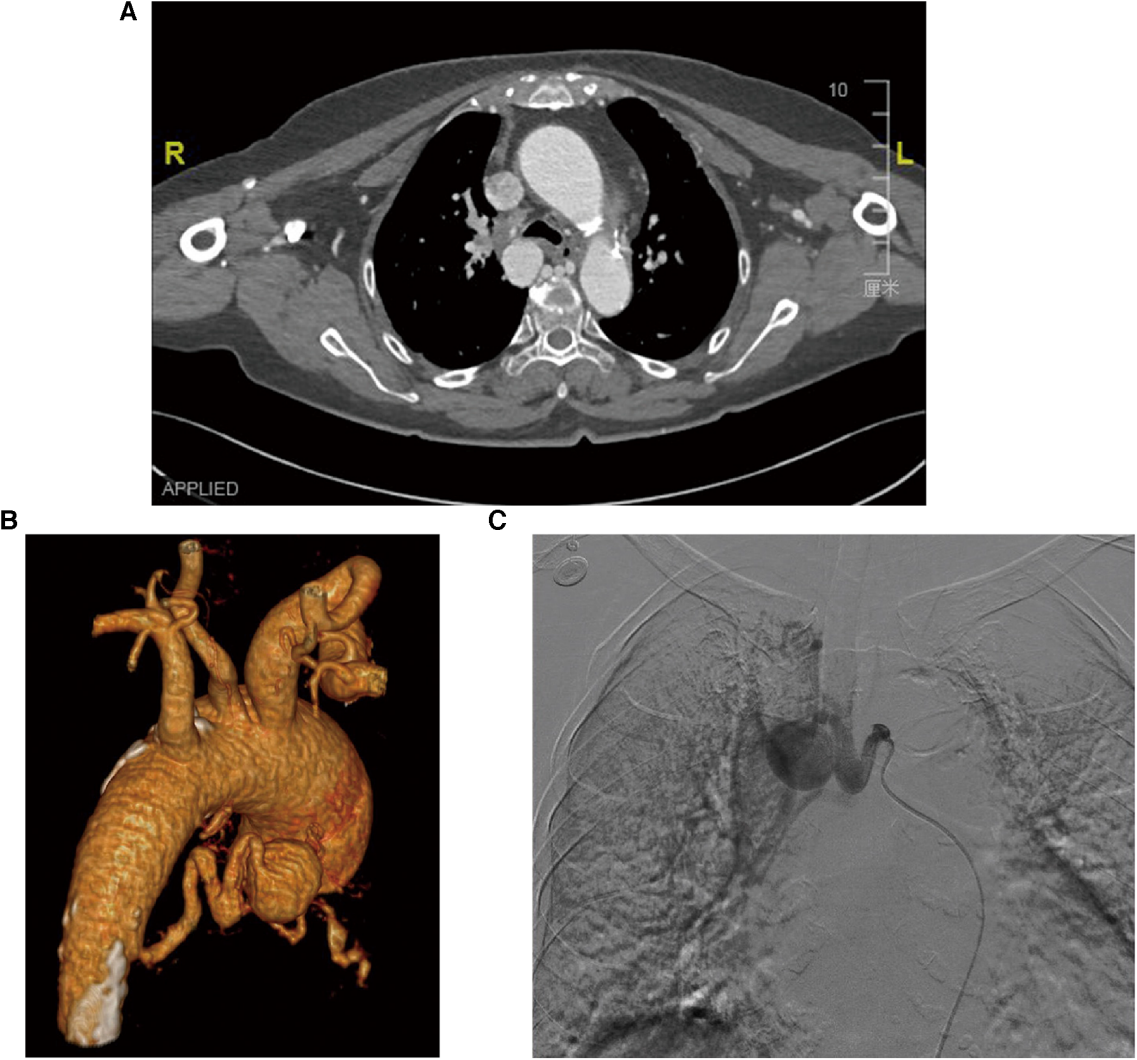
Diagnostic images of the patient with BAA. (A) The CT scan image of the chest shows an abnormally enhanced lesion in the mediastinum and dilated bronchial arteries near the descending aorta, but no entrapment or hemopneumothorax. (B) Three-dimensional reconstruction of the CT angiographic model shows a 25-mm-diameter BAA from the descending aorta with a dilated and tortuous inflow vessel. (C) Selective bronchial arteriography shows that the aneurysm was fed by a bronchial artery dilated by the descending aorta.
